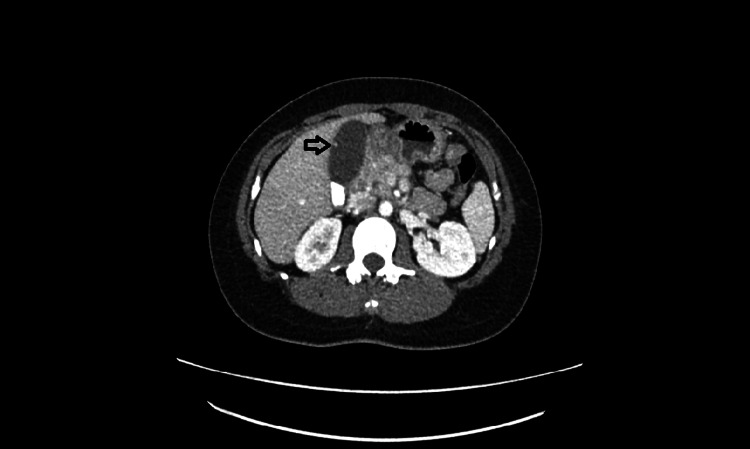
Small gallbladder polyp seen on computed tomography.
